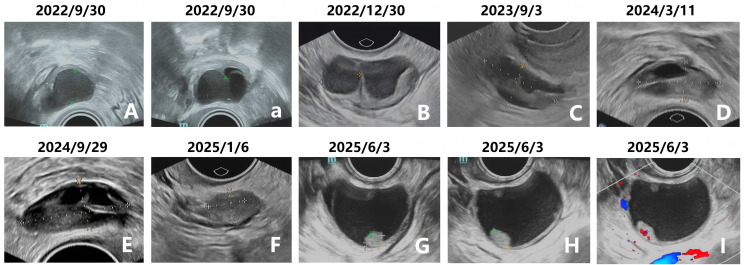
Serial transvaginal ultrasound monitoring of ovarian endometriomas during dienogest therapy. (A) Baseline ultrasound (September 2022) shows an endometrioma in the right ovary. (a) Baseline ultrasound (September 2022) shows another endometrioma in the right ovary. (B) Significant regression of cysts after 3 months of treatment (December, 2022). (C) Complete sonographic resolution after 12 months of continuous therapy (September, 2023). (D) No evidence of recurrence on follow-up ultrasound (March, 2024). (E) Continued absence of recurrence (September, 2024). (F) Recurrence of a right ovarian endometrioma at 27 months of therapy (January, 2025). (G–I) Rapid progression to a complex cyst with papillary projections and internal vascularity within 5 months (June, 2025).
